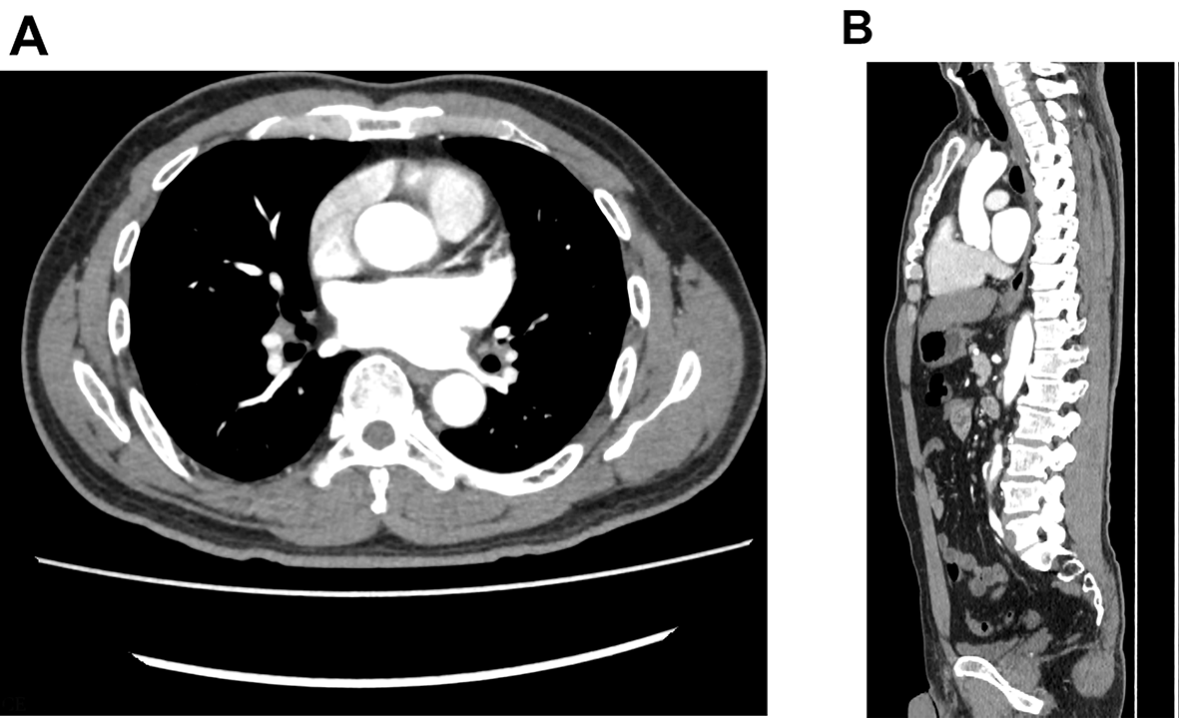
(A) and (B): Follow-up CTA at 6 months demonstrating complete resolution of the previous thrombus
